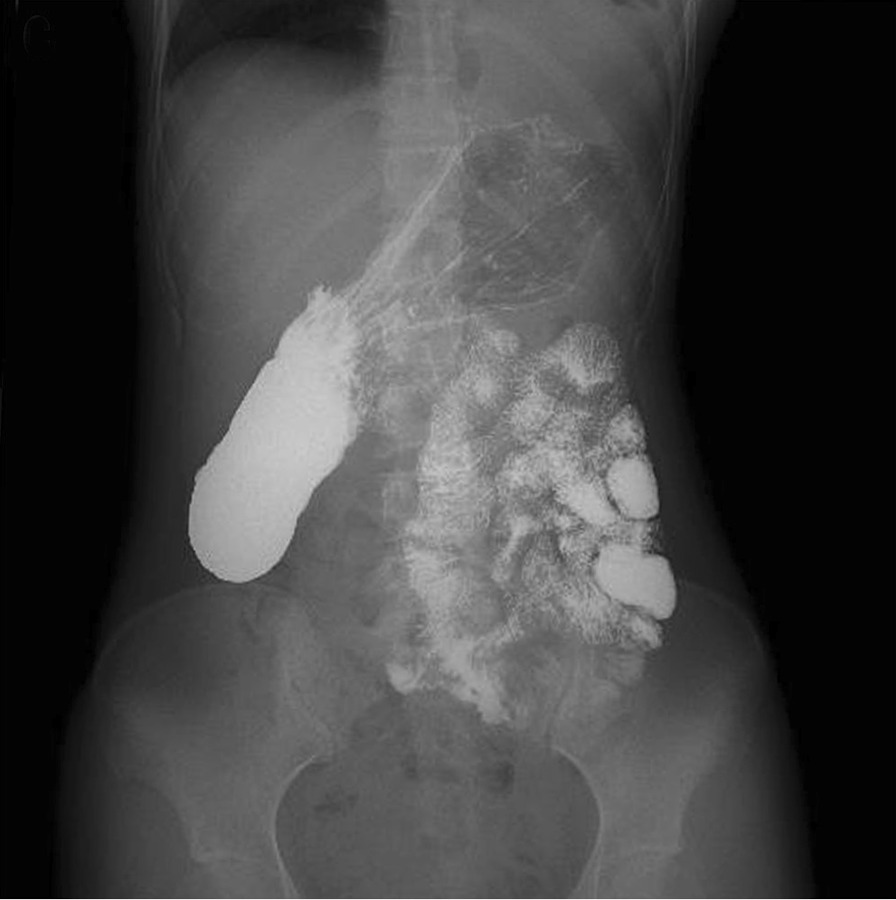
X-ray with soluble iodine contrast at the 1-month follow-up showing the upper gastrointestinal tract and that the repair was satisfactory
